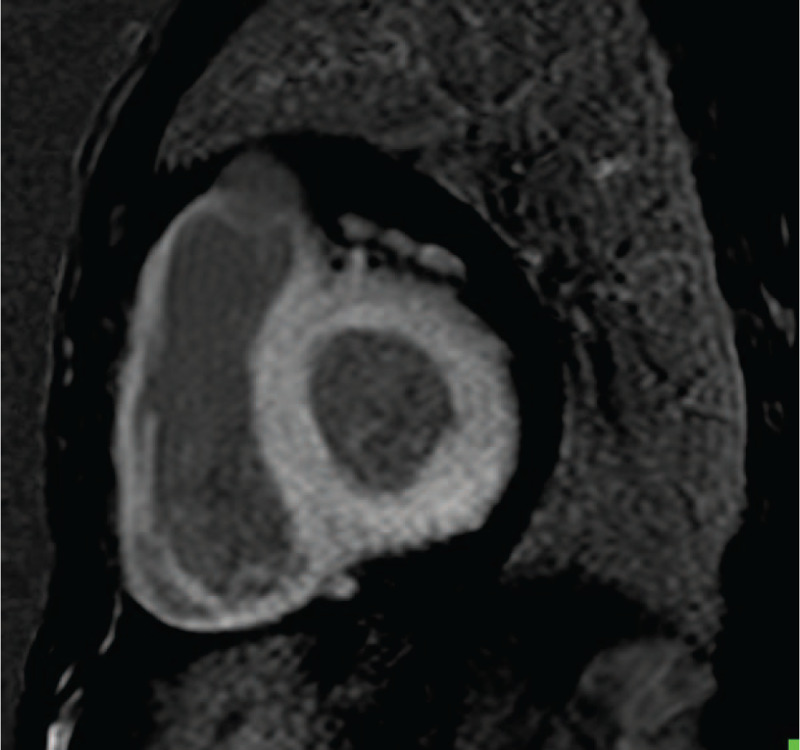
CMR. Cardiac magnetic resonance imaging (CMR) showed a diffuse transmural late gadolinium enhancement (LGE) in ventricular walls. Cardiac magnetic resonance imaging showed a diffused transmural LGE in both ventricular walls which suggested cardiac amyloidosis.
